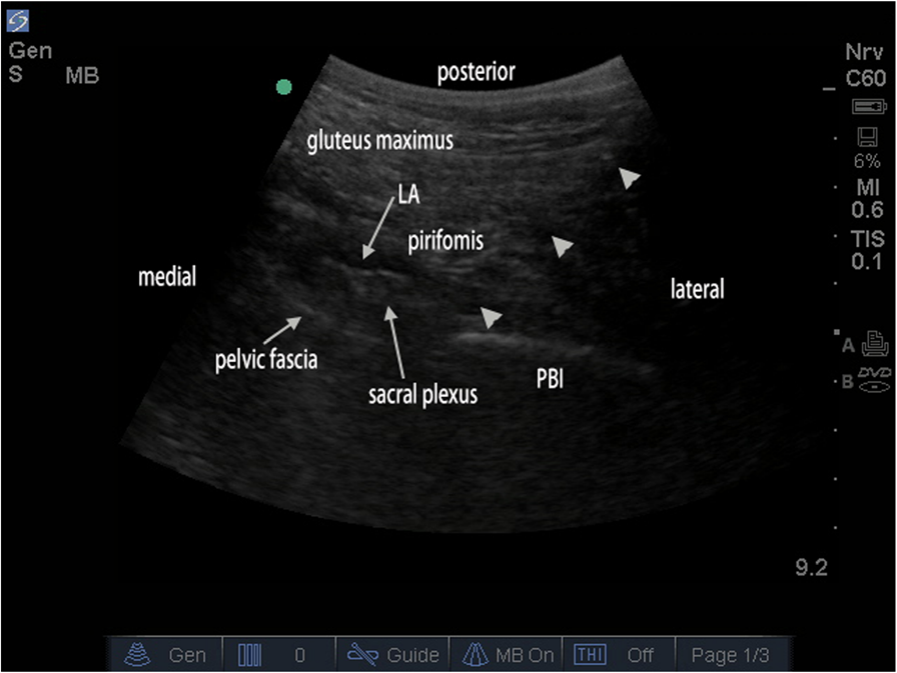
Ultrasonography of the sacral plexus bathing in local anesthetic deep to the pirifomis muscle and medial to the PBI. (LA = Local anesthetic, PBI = posterior border of ischium, triangle indicates the needle)
